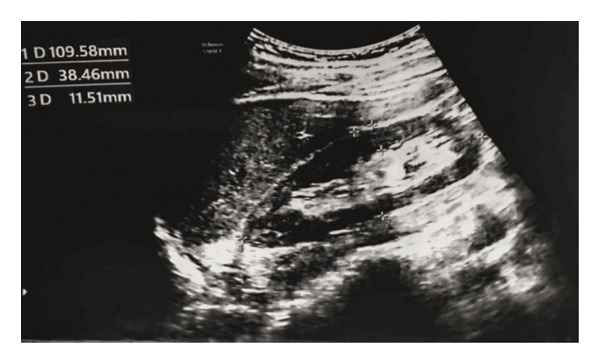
Renal ultrasound showing preserved corticomedullary differentiation.
